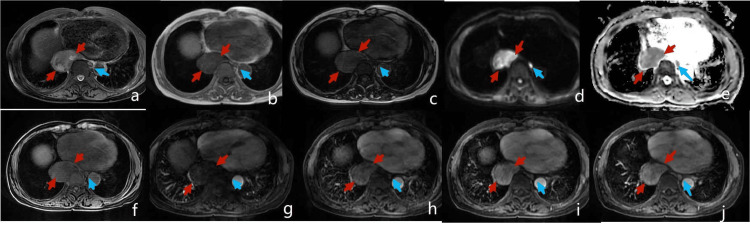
MRI of the mediastinum (a) T2-weighted imaging (T2WI). (b-c) T1-weighted imaging (T1WI) in and out of phase. (d-e) Diffusion-weighted imaging (DWI) and apparent diffusion coefficient (ADC) image. (f-j) T1WI of pre-enhancement. (f) Dynamic enhancement. (g-j) delayed enhancement.
